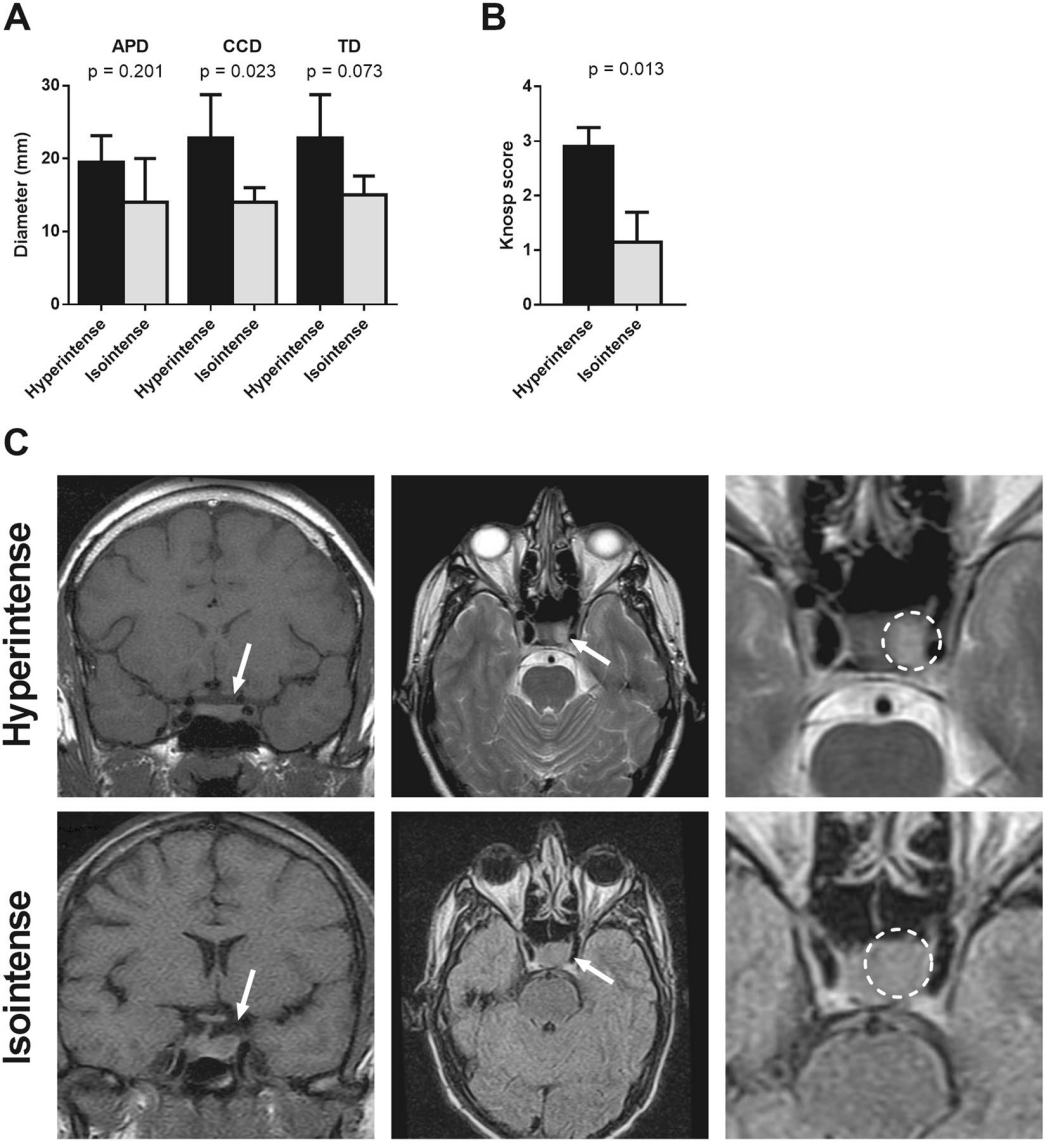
Association between T2-signal and the diameter (A) and Knosp score (B) of somatotropinomas. The diameter of GH secreting adenomas was measured in millimetres. APD: anteroposterior diameter; TD: transverse diameter; CCD: craniocaudal diameter. Data represent median ± interquartile range. (C) Classification of T2-weighted signal of GH secreting pituitary adenomas: T2-hyperintense adenomas, upper-panel and T2-isointense adenomas, lower-panel. Tumour region was indicated with an arrow in coronal MRI (left), and in T2-weighted axial MRI (middle), the tumour area was indicated by a circle in right panel.
